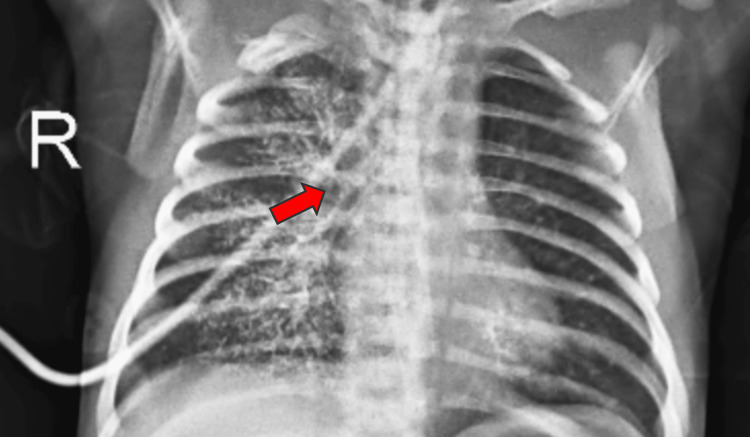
Chest X-ray taken one minute after giving contrast, showing the presence of contrast material in the right lung (red arrow)
